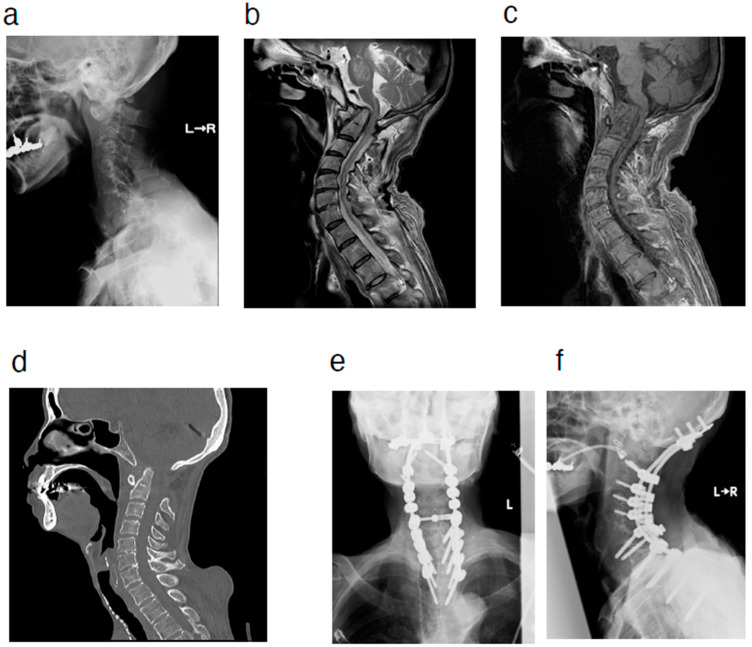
(a) Lateral view of radiographic image at readmission. (b,c) MRI at readmission. Sagittal view of the T2-weighted (b) and T1-weighted (c) images. (d) CT imaging at readmission. Sagittal view. (e,f) Radiographic images after reoperation. A-P view (e) and lateral view (f). MRI = magnetic resonance imaging. CT = computed tomography.
